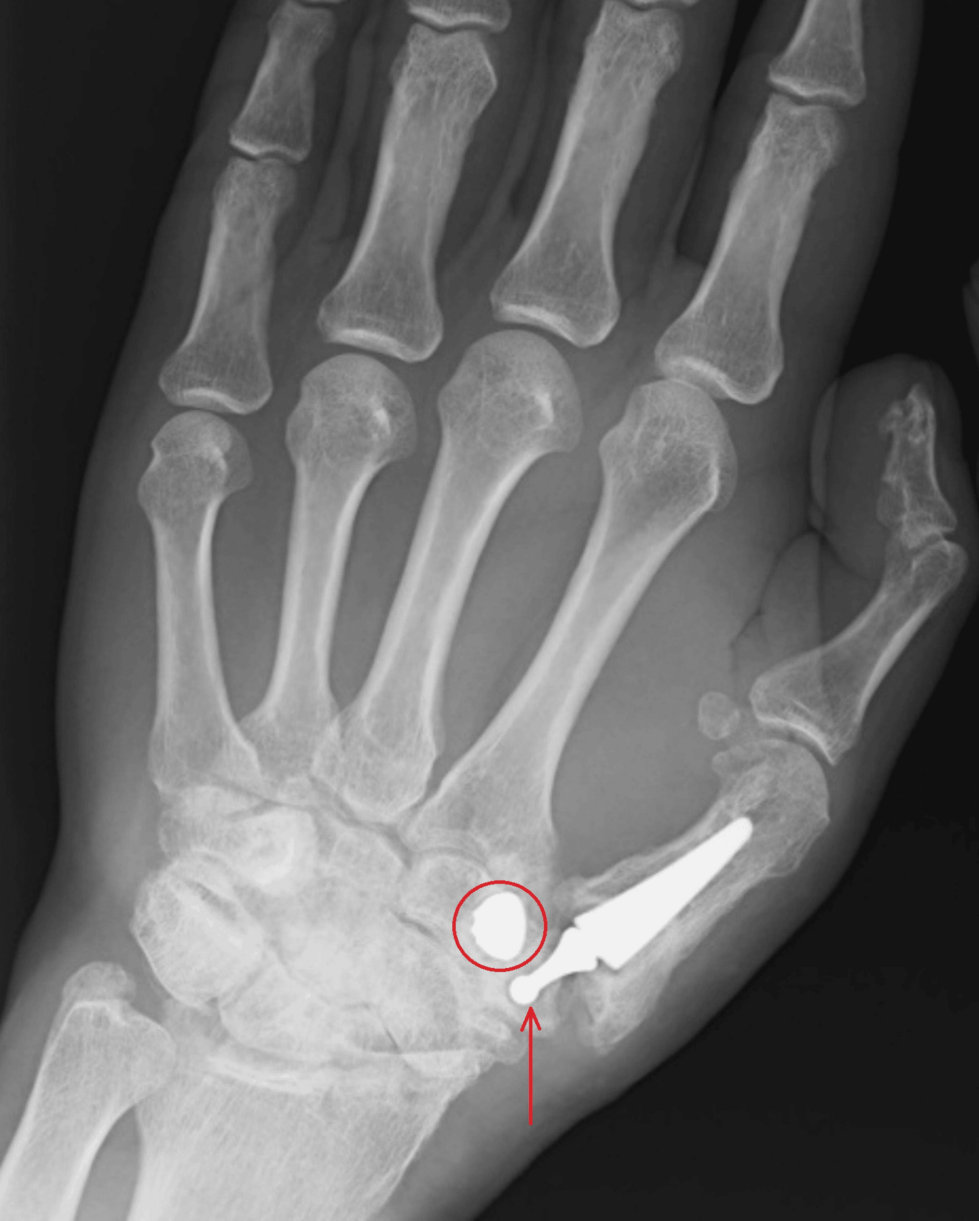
X-ray in A-P projection obtained three years after surgery The figure shows the dislocation of the implanted endoprosthesis elements. The red arrowhead marks the dislocated head of the double mobile CMC thumb prosthesis. The open circle marks the metal acetabular shell. A-P: anterior-to-posterior; CMC: carpometacarpal
